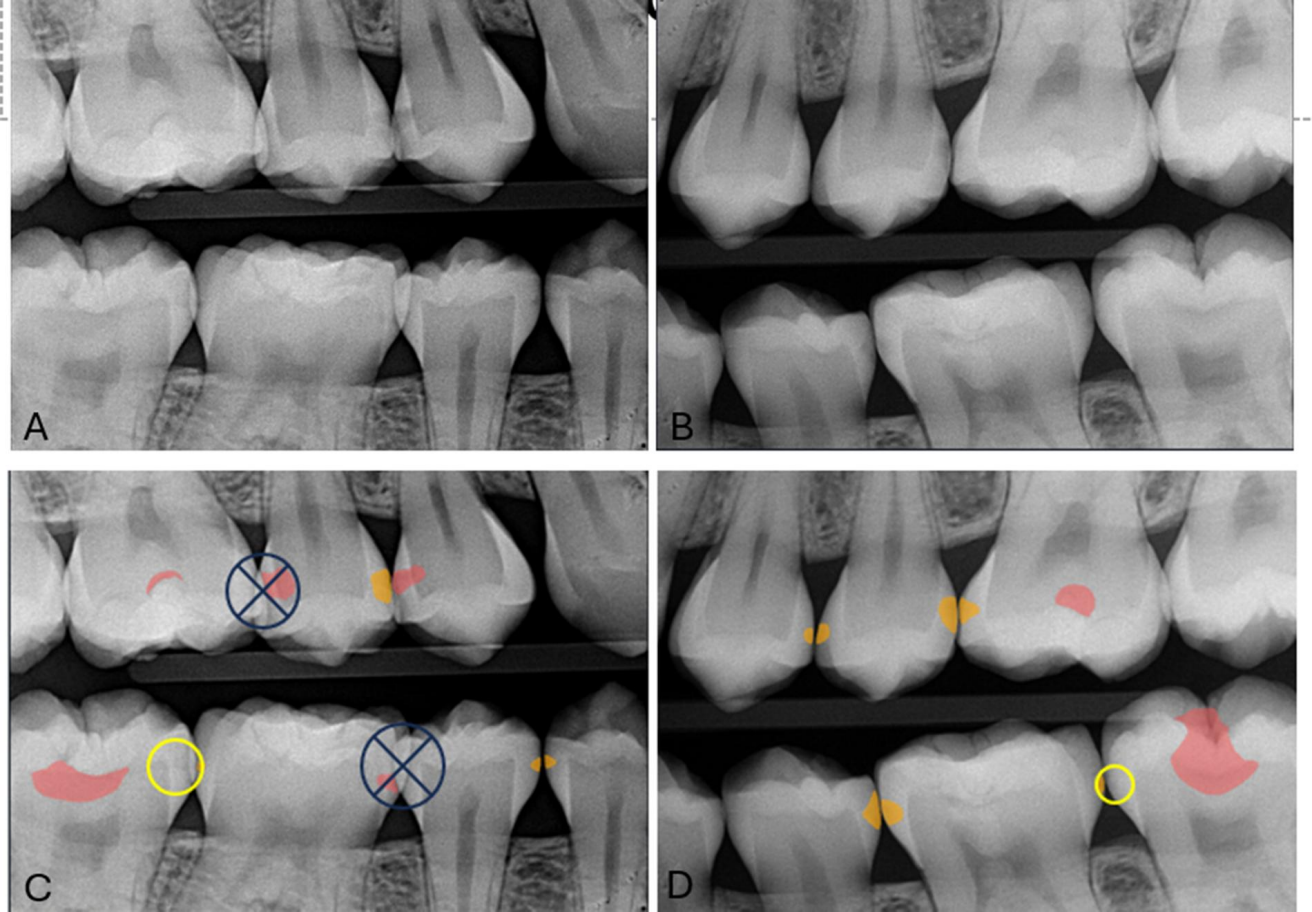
Bitewing radiographs (A,B) without OCA interpretation. Images (C,D) show OCA program applied. “early enamel caries” caries labeled in orange and “treatable” caries labeled in red. The yellow circle indicates caries false negative and the X indicates surfaces with overlap that did not qualify for consideration.
